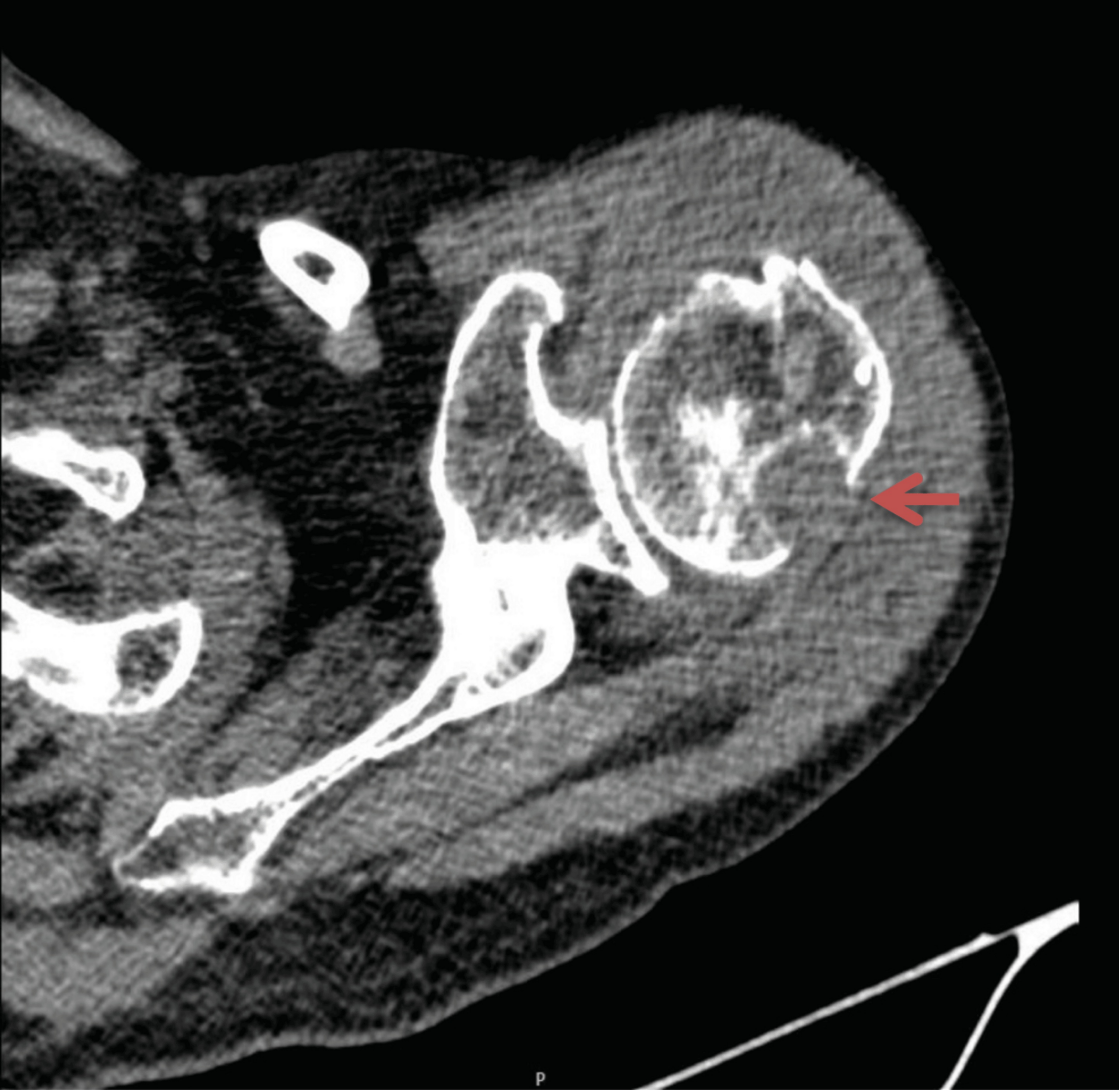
Right Shoulder CT scan demonstrating marked inflammatory changes Red arrow: Posterior aspect of humeral head with substantial erosion
